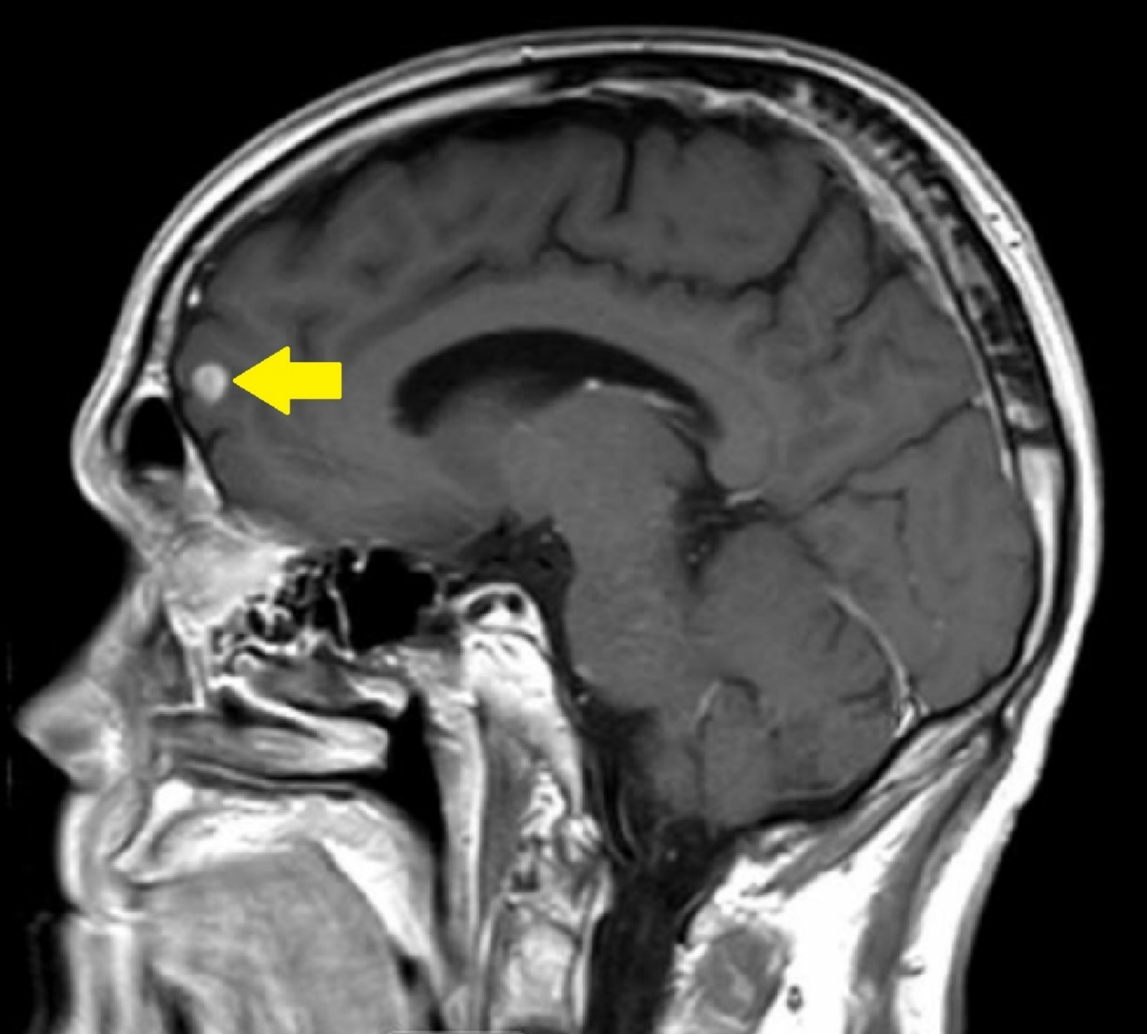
Left Frontal Lobe Metastasis Pre-Treatment Sagittal Brain Magnetic Resonance Imaging (MRI).
